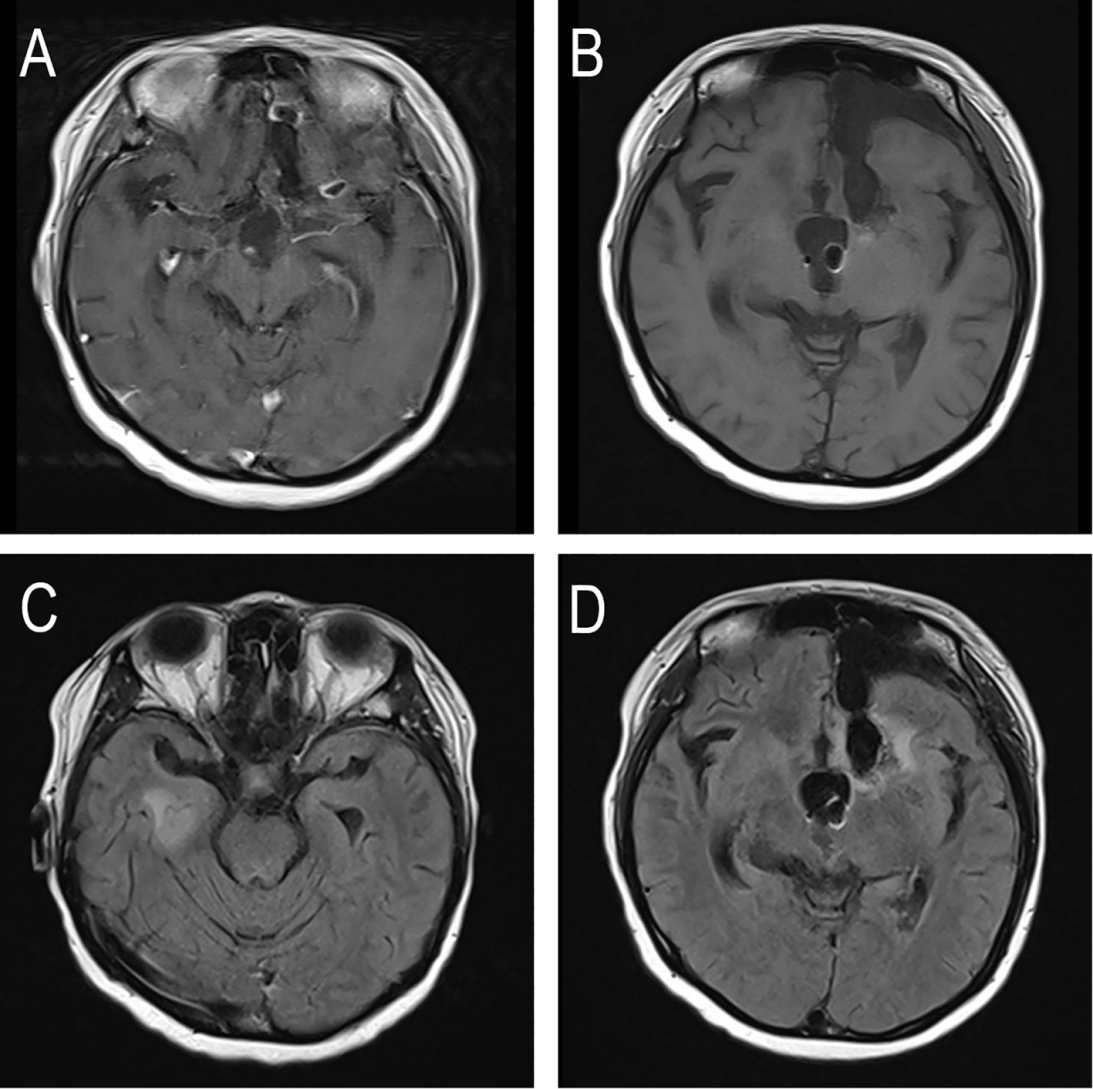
The patient’s MRI after re-radiotherapy. (A, B) T1-weighted contrast-enhanced MRI showing linear enhancement at the surgical margin and in the inferior horn of the right lateral ventricle. (C, D) T2-FLAIR MRI showing linear enhancement at the surgical margin and in the inferior horn of the right lateral ventricle.
